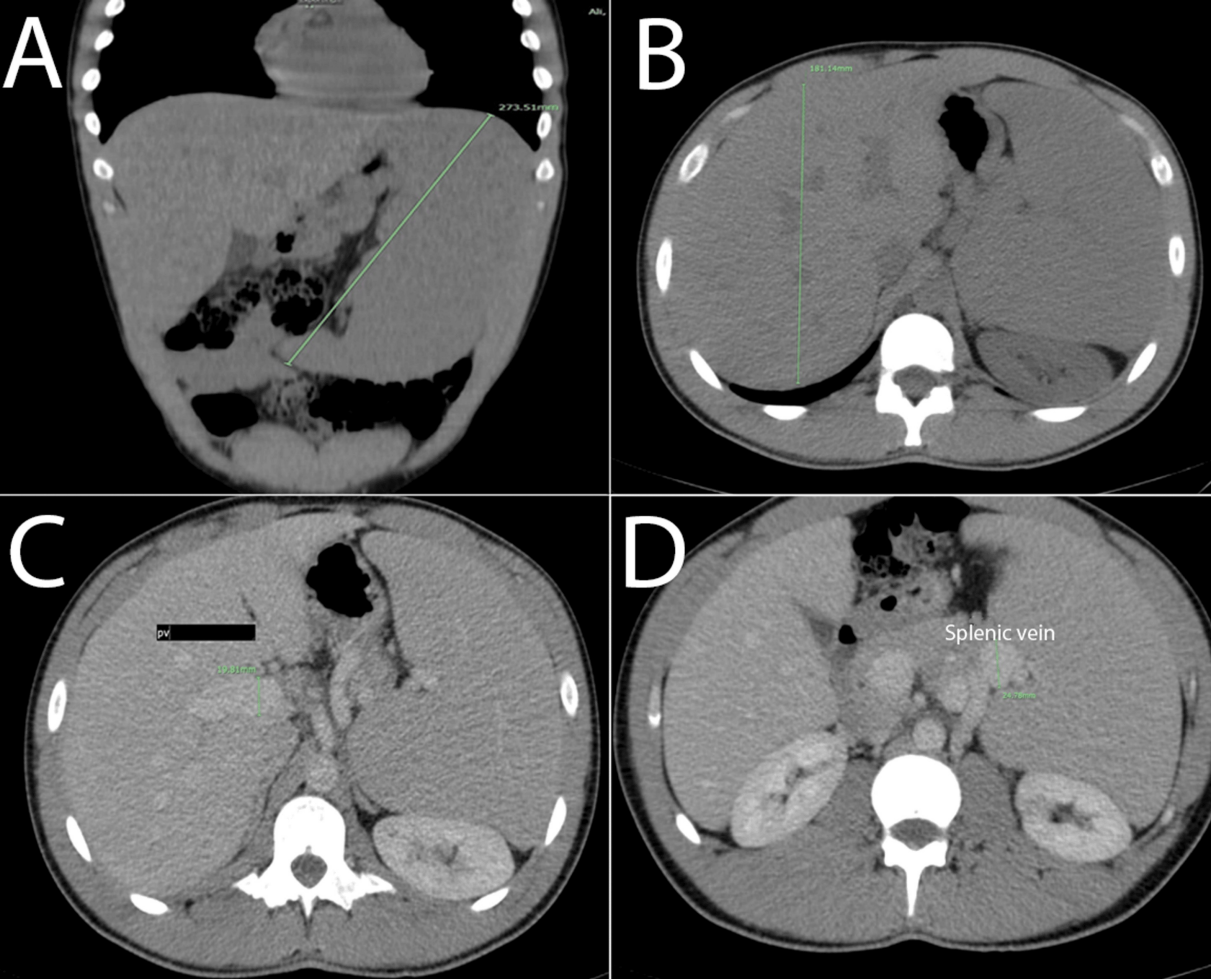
Abdominal CT scan Abdominal CT scan reveals splenomegaly measuring 27 cm craniocaudally (A), hepatomegaly measuring 18.5 cm in anteroposterior diameter (B), dilatation of the portal vein and its confluent measuring 2 cm in anteroposterior diameter (C), and dilatation of the splenic vein reaching 2.5 cm in anteroposterior diameter (D)
